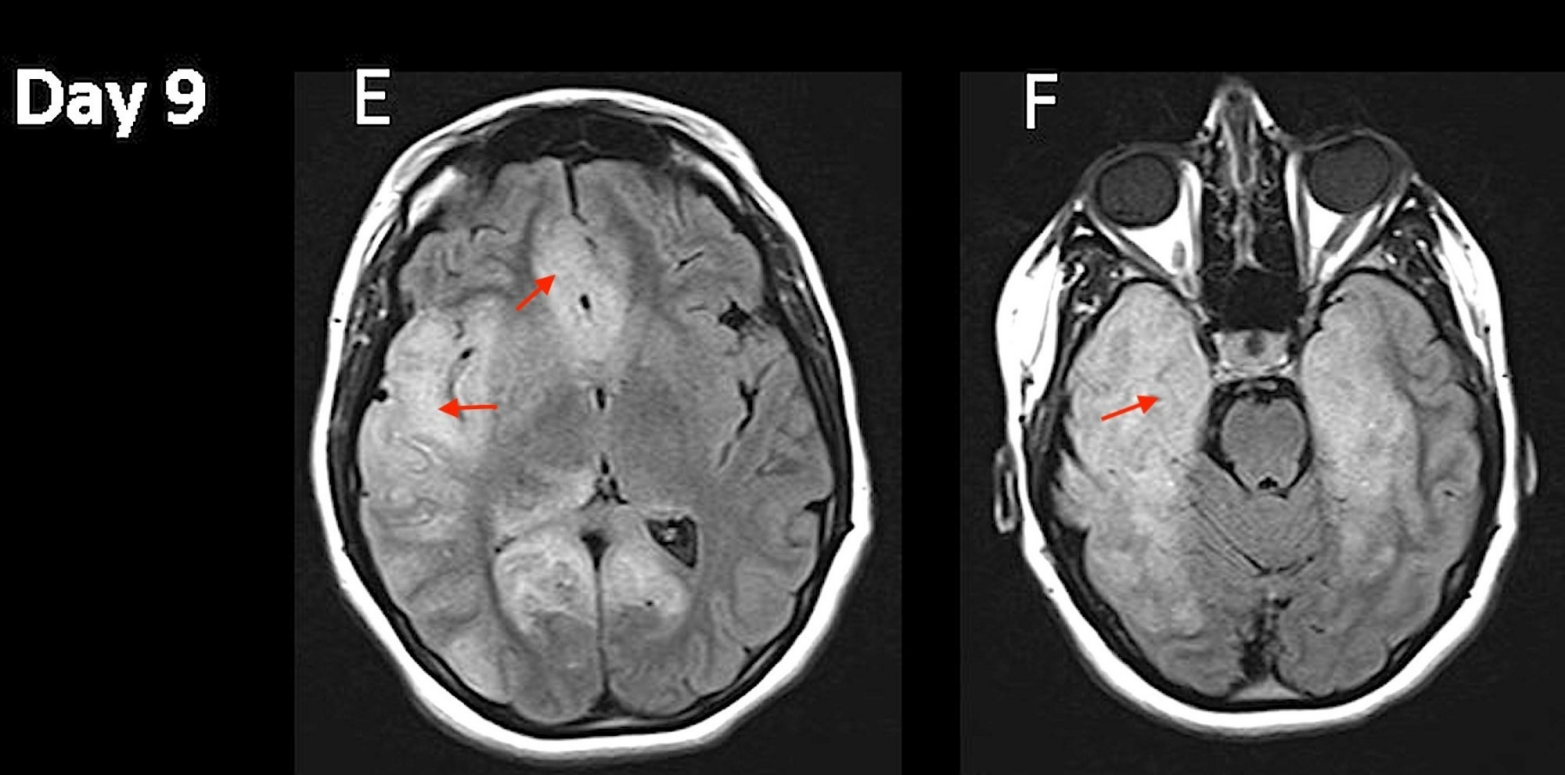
Magnetic resonance imaging (MRI). Hospital day 9 fluid attenuated inversion recovery (FLAIR) changes evolution (E and F).
